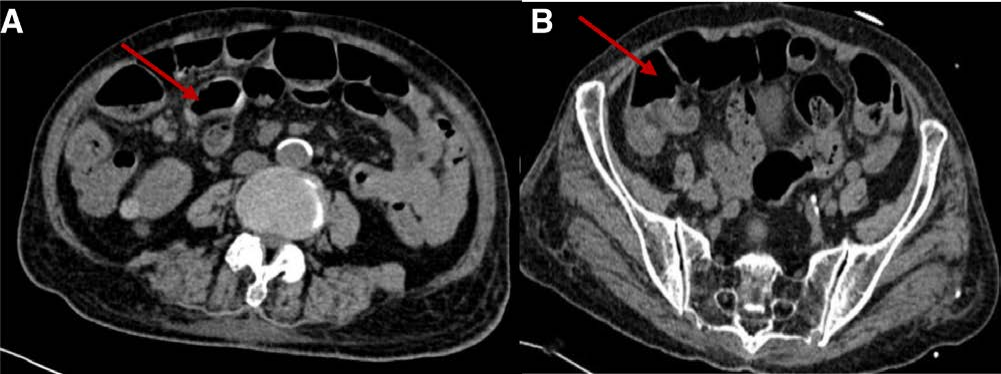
Abdominal CT scan views showing partial dilated small intestine (A) and colon (B) with effusion and multiple fluid levels (arrows). CTA = computed tomography angiogram.
